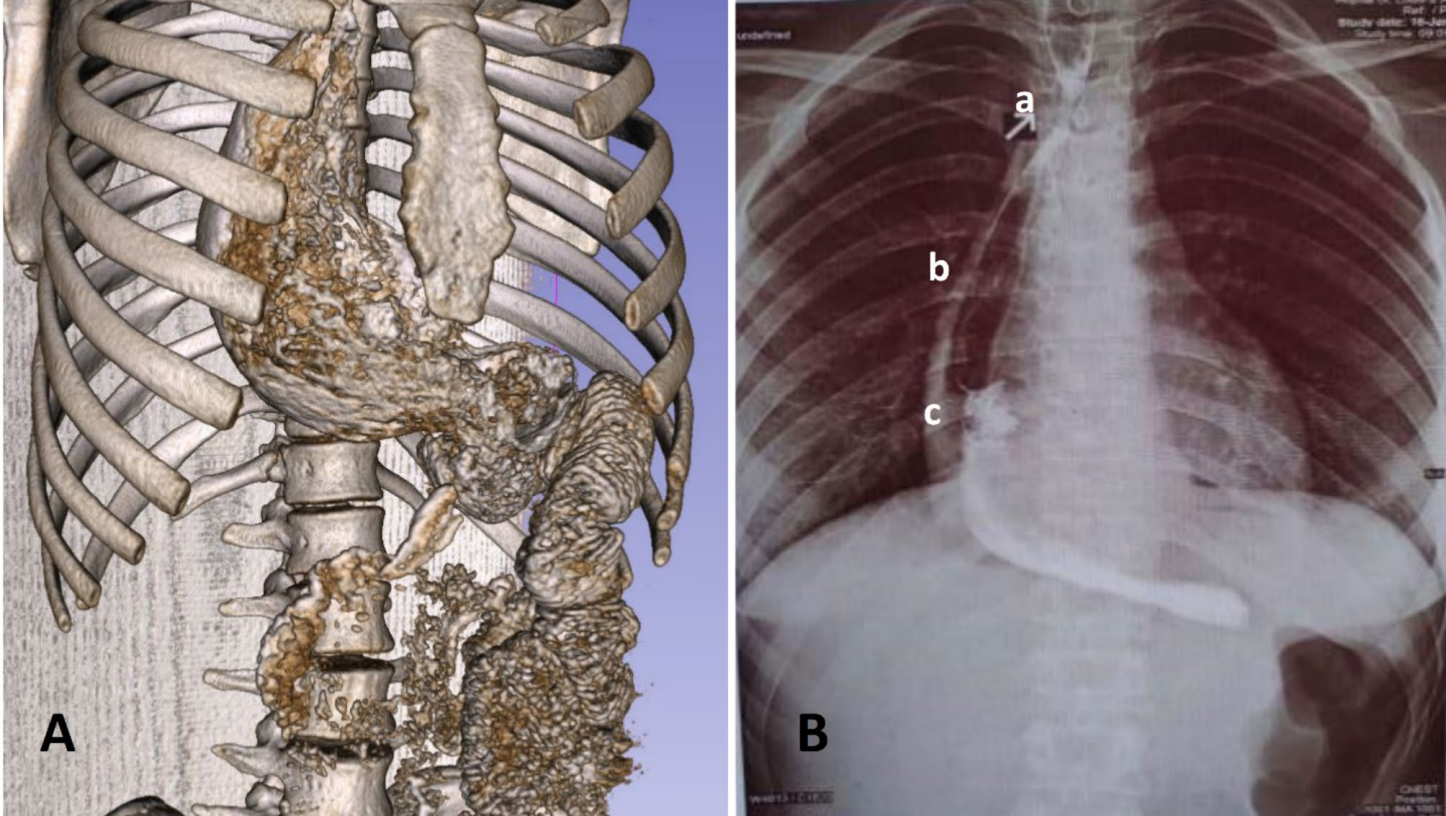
A: 3-dimentional view of the multi-Slice computerized tomography scan with barium swallowing after the new procedure demonstrating a good passage of contrast agent through the anastomosis. B: Chest X-ray with barium swallowing demonstrates a stricture in the esophago-gastric anastomosis (a), the pulled-up stomach (b) and the gastro-jejunal anastomosis (c)
